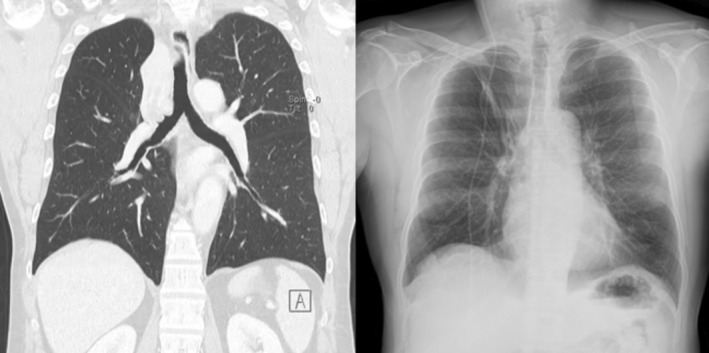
Radiological comparison before and after bronchoscopic treatment. Left: Coronal contrast‐enhanced chest CT demonstrating complete right upper lobe atelectasis due to obstruction of the right upper lobar bronchus. Right: Post‐procedural chest X‐ray demonstrating re‐expansion of the right upper lobe after rigid bronchoscopic debulking.
